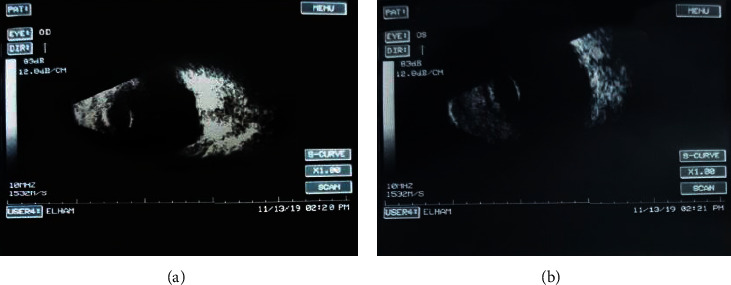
Ocular B-scan ultrasound of the right (a) and left (b) eyes. The ultrasound revealed an axial length of 17 mm bilaterally with no other remarkable findings.
